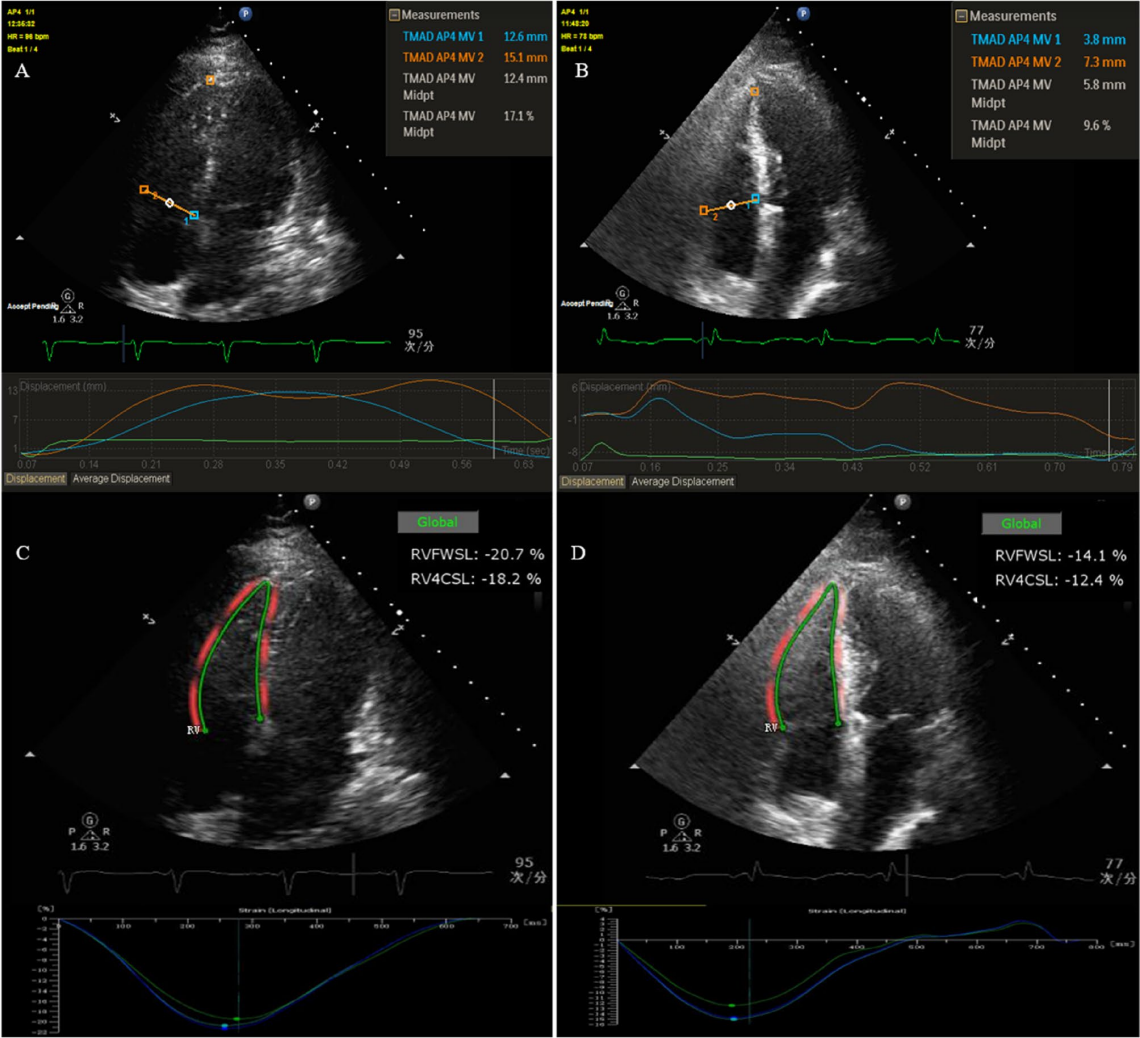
Speckle tracking echocardiography (STE) images of septic patients with or without right ventricular dysfunction (RVD). A Four-chamber view of the right ventricular (RV) tissue motion annular displacement (RVTMAD) measurement of one patient with normal RV function. B Four-chamber view of the RV tissue motion annular displacement (RVTMAD) measurement of one patient with right ventricular dysfunction (RVD). C four-chamber view of the RV longitudinal strain (RVLS) measurement of one patient with normal RV function. D Four-chamber view of the RV longitudinal strain (RVLS) measurement of one patient with RV dysfunction (RVD)
